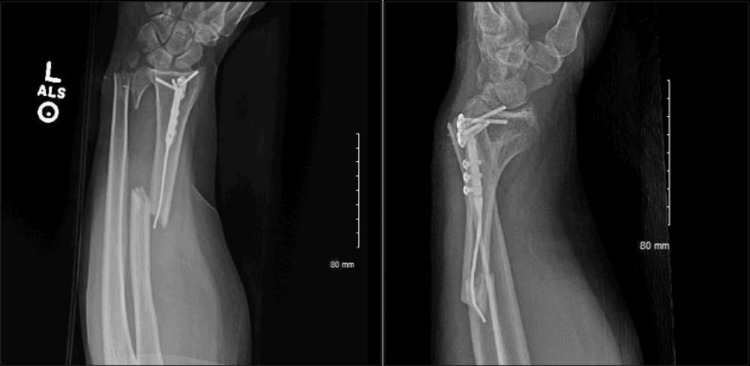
Anteroposterior (A) and lateral (B) X-ray films demonstrating an extra-articular peri-implant fracture of the left distal radius, along with a concomitant displaced intra-articular comminuted fracture of the distal ulna
